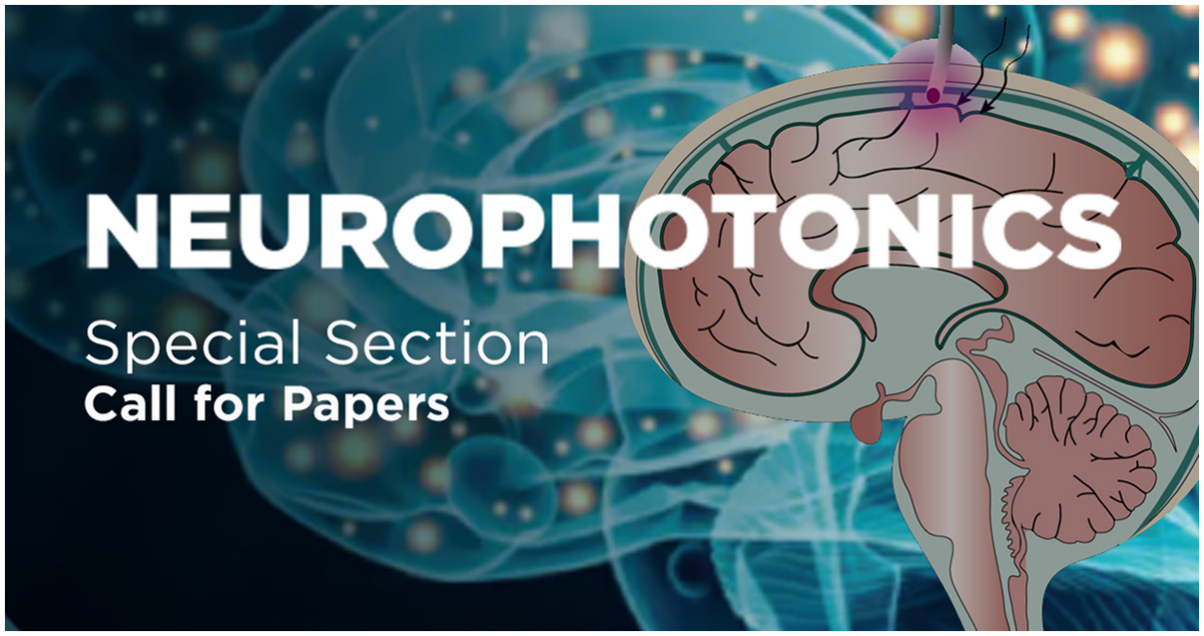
Do you combine optics and photonics with ultrasound, transparent electronics, MRI, or other technologies to study the brain? Submit to the Special Section on Hybrid Photonic/X Neurointerfaces!
